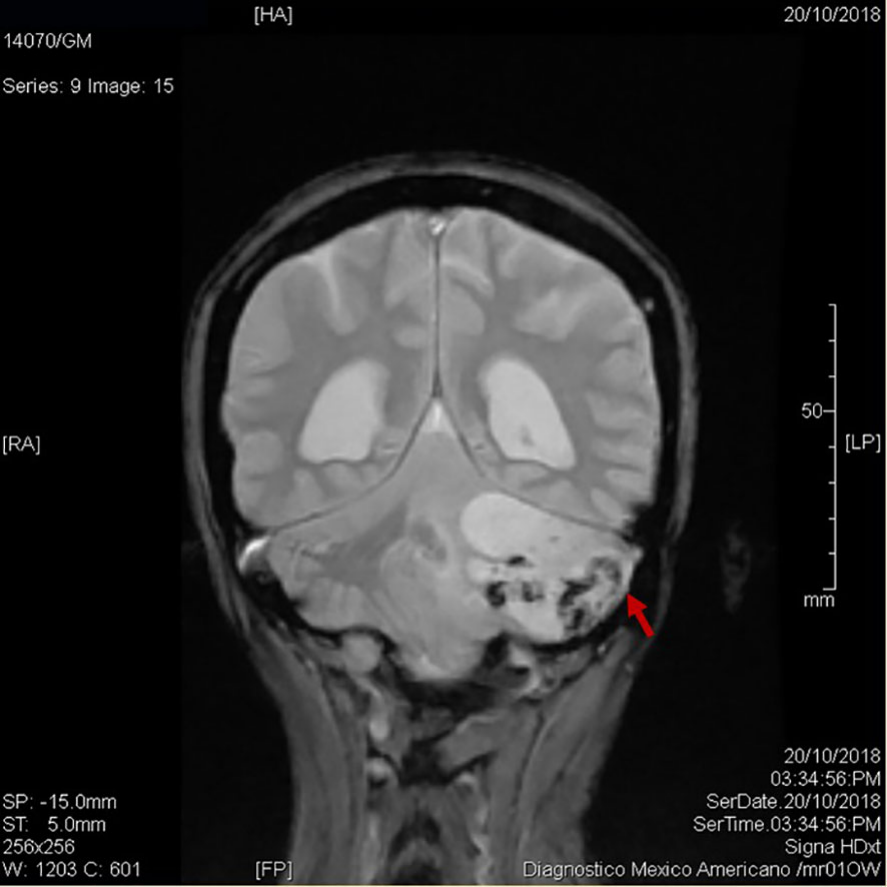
Subsequent MRI of brain cerebellar metastasis. Coronal view: showing an infratentorial mass (36 x 39 x 48 mm) in the left cerebellar lobe (red arrow), leading to tonsillar herniation, compression of the fourth ventricle, and secondary supratentorial ventriculomegaly.
